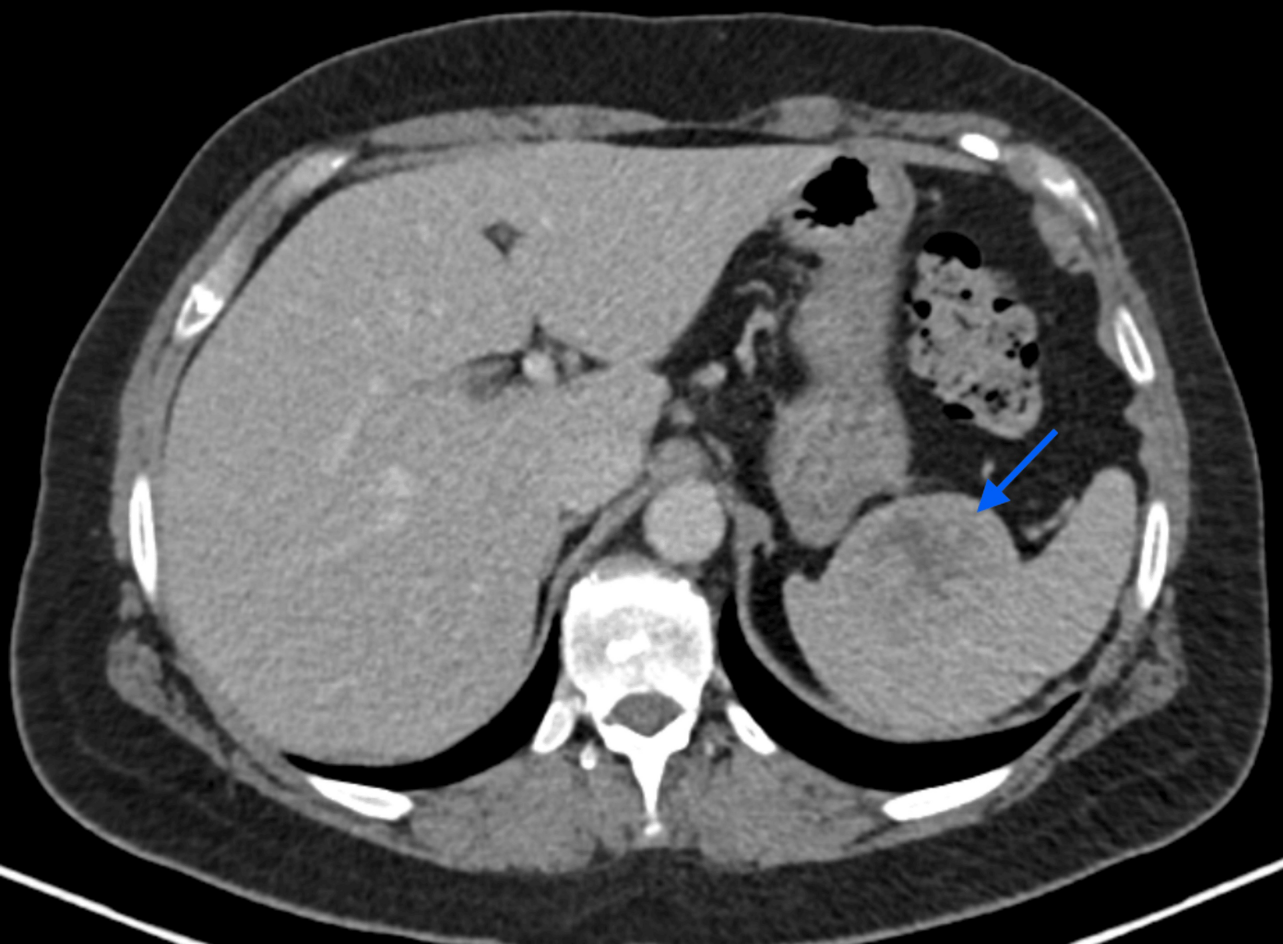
Patient's abdominal axial CT scan Non-contrast-enhancing 55 mm heterogeneous nodule in the spleen signaled with a blue arrow.
